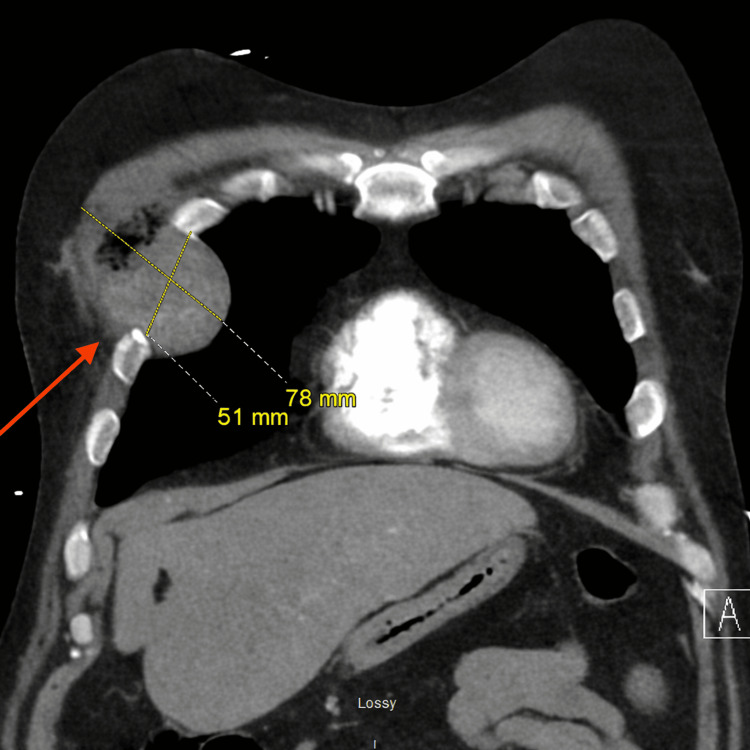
Chest CT scan with IV contrast. Coronal CT reconstruction demonstrating a lobulated soft tissue mass extending from the thoracic cavity through the right anterior chest wall into the retroareolar/subareolar region (red arrow), measuring approximately 51 × 78 mm in greatest dimensions.
